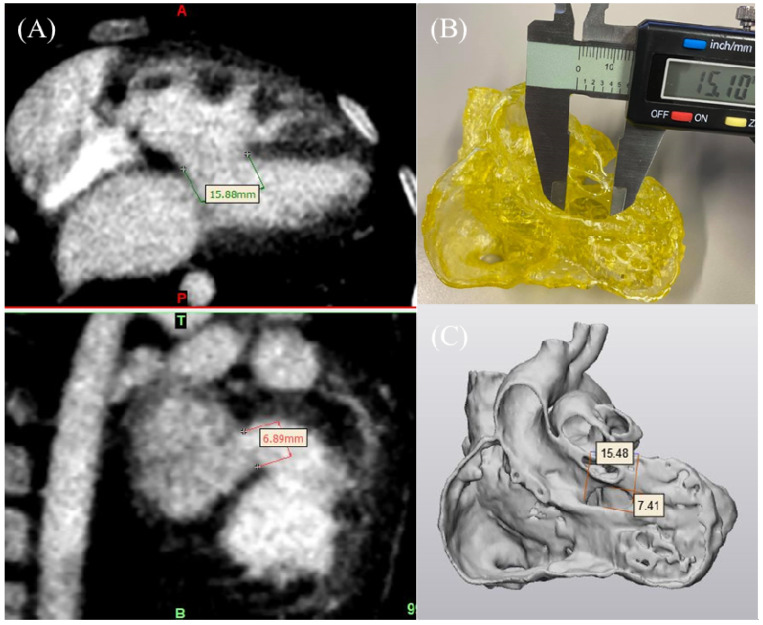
3D model accuracy evaluation. (A) CT imaging data in coronal and sagittal views to measure the VSD in Mimics. (B) Measurement of the VSD in the 3D-printed model using a digital calliper. (C) STL file measurement of the VSD in 3-Matic. VSD-ventricular septal defect.
